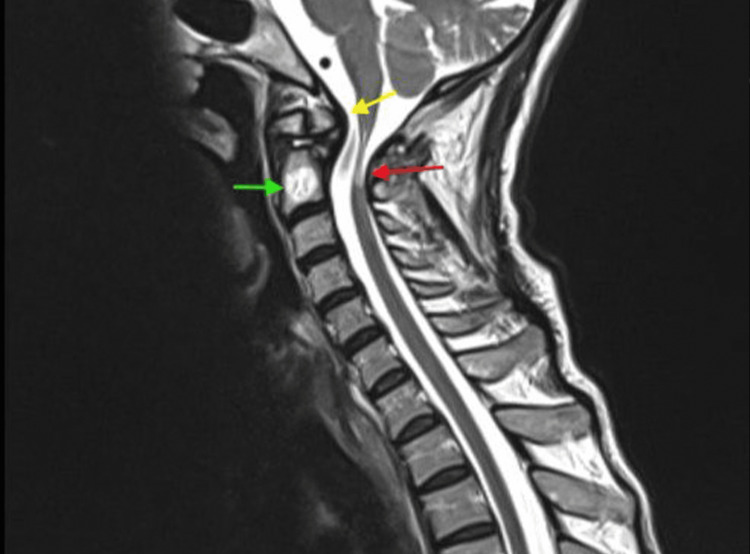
Magnetic resonance imaging (MRI) The MRI scan shows a thickened tectorial membrane (yellow arrow), marked focal atrophy of the most proximal portion of the cervical cord at the CV1 and CV2 levels, displaying an intramedullary T2 hyperintense signal (red arrow), significant spinal cord compression at the same level, and a vertebral body hemangioma noted at the CV2 vertebral body (green arrow).
